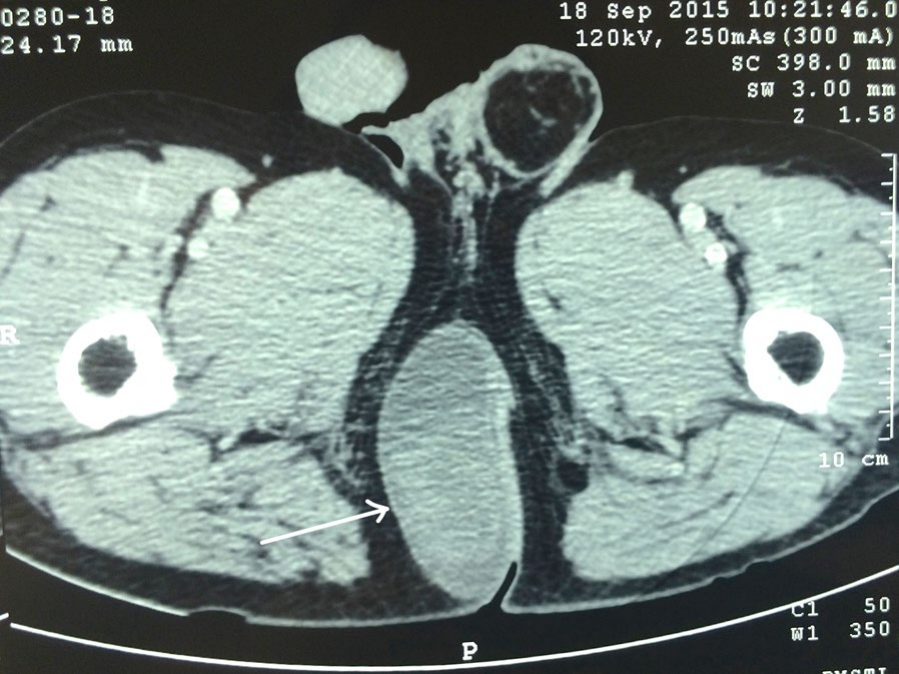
Computed tomography showing the perineal mass, partially enhanced after injection of contrast medium (*arrow*)
